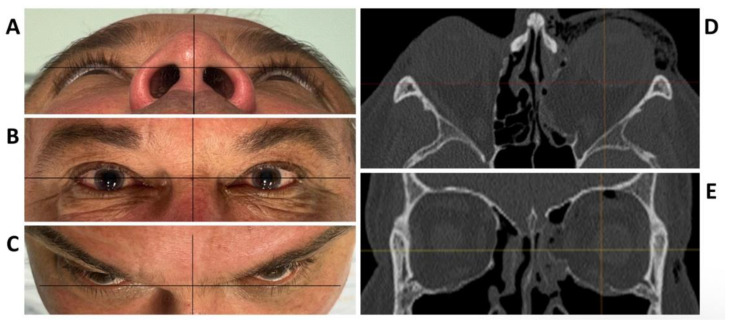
Clinical photographs of the patient showing an enophthalmos in the perspective from below (A), and above (C), while there is no hyper- or hypoglobe present as visible in the frontal view (B). Axial (D) and coronal (E) plane of the pre-operative CT scan displaying the traumatic defect of the medial wall and floor of the left orbit.
